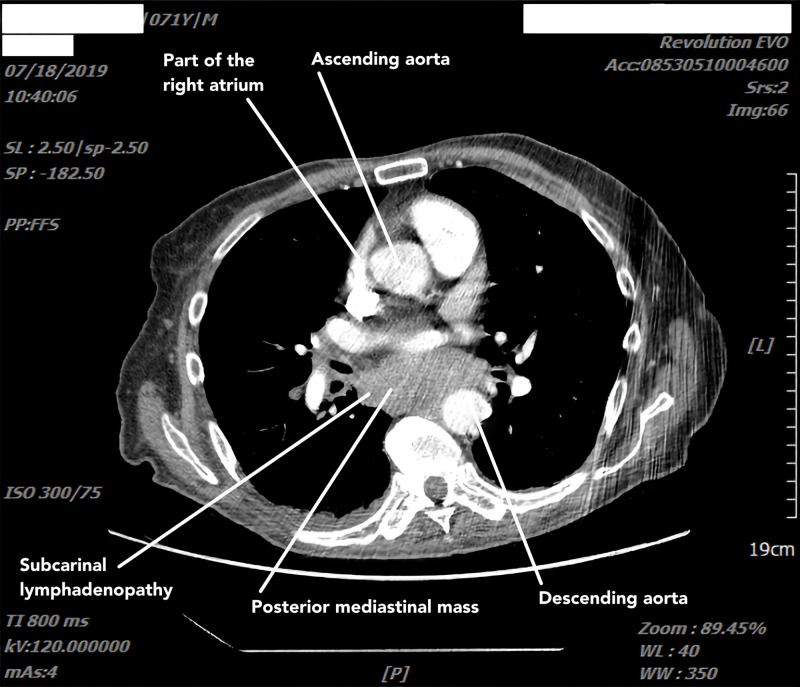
Chest CT scan with contrast showing posterior mediastinal mass and significantly enlarged lymph nodes in right and left paratracheal and subcarinal lymph nodes.
